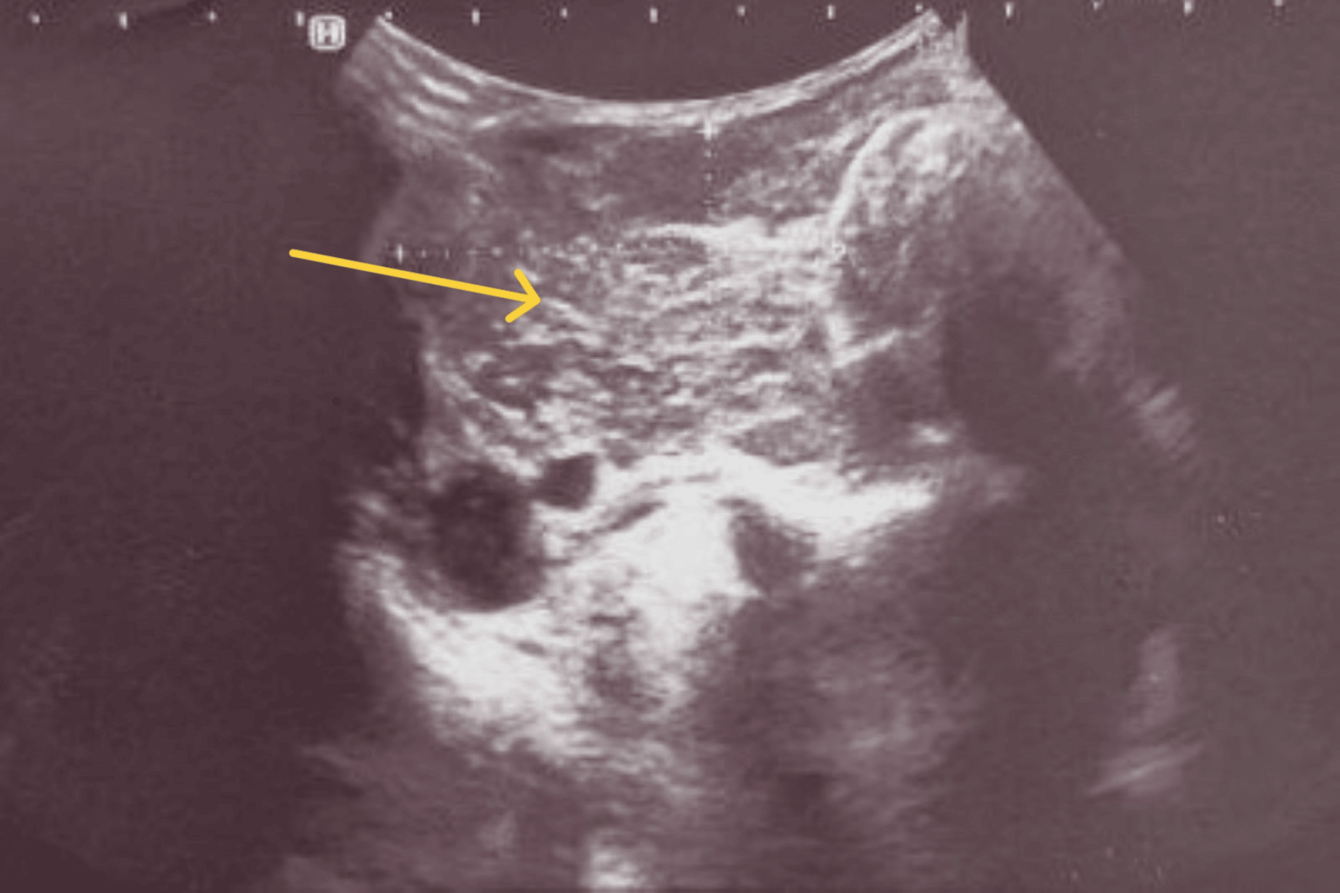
Cervical ultrasonography demonstrated a large substernal goiter with a markedly heterogeneous, multinodular architecture (yellow arrow).
